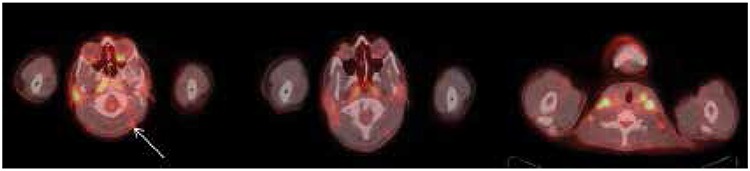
Subcutaneous mildly fluorodeoxyglucose avid lesions are seen in the occipital and upper para-spinal regions, SUVmax: 2.5 (arrow)
